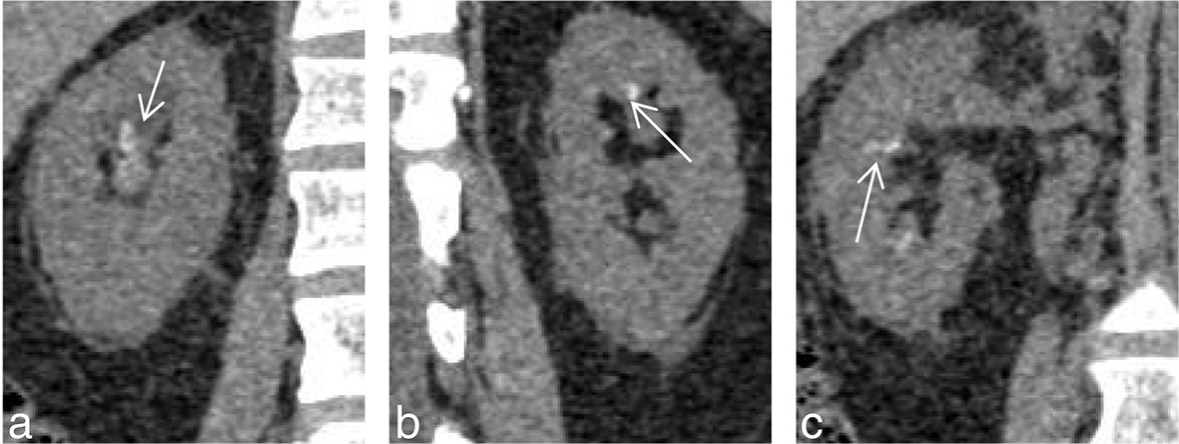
(a–c) (Same patient as in Figure 3) Coronal reformats of CT images showing papillary calcification in both kidneys (arrows).
